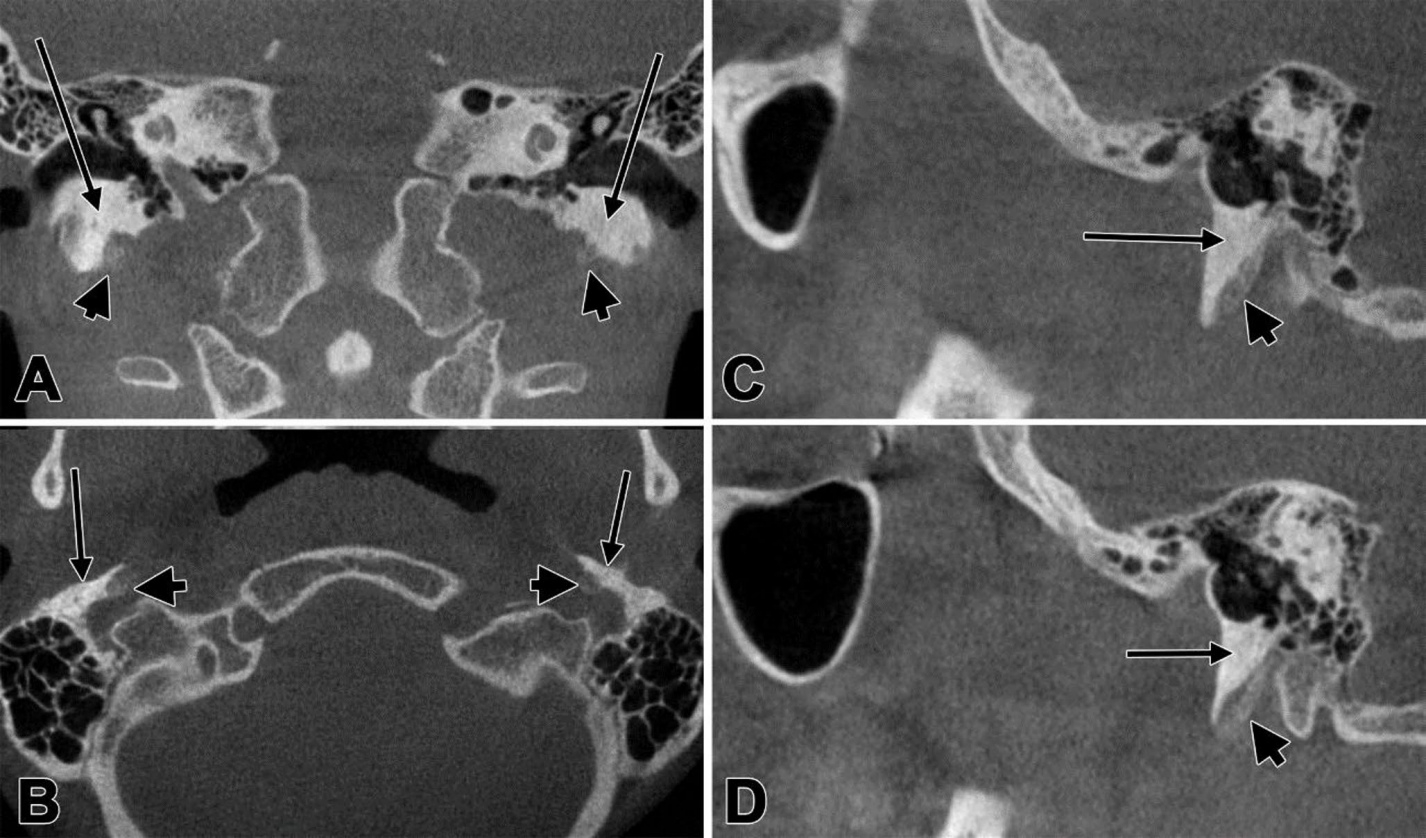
Bilateral CBCT evidence of low-density tympanohyal segments (arrowheads) beneath the vaginal processes of the tympanic plates (arrows). A coronal slice, anterior view; B axial slice, inferior view; C Sagittal slice, left side, lateral view; D Sagittal slice, right side, medial view
